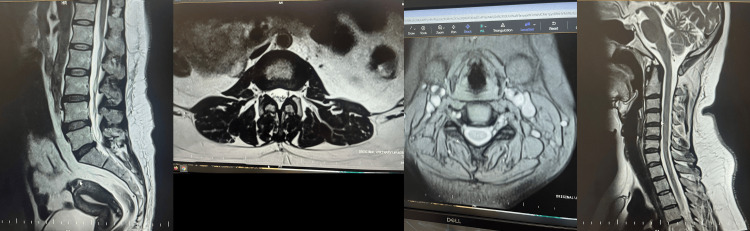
T1-weighted axial and sagittal sequences No dorsal nerve root thickening was noted in the cervical and dorsal lumbar region.
